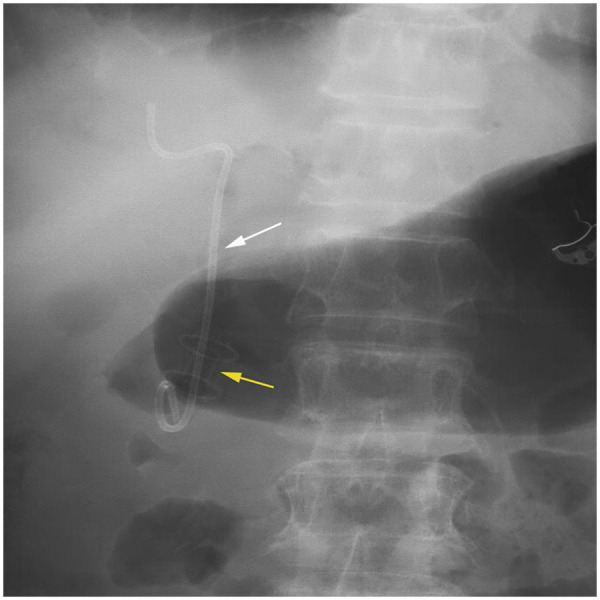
Abdominal X-ray confirming successful placement of a coaxial double-pigtail plastic stent (white arrow) within the LAMS (yellow arrow). The pigtail curls are visible at both ends of the plastic stent.
